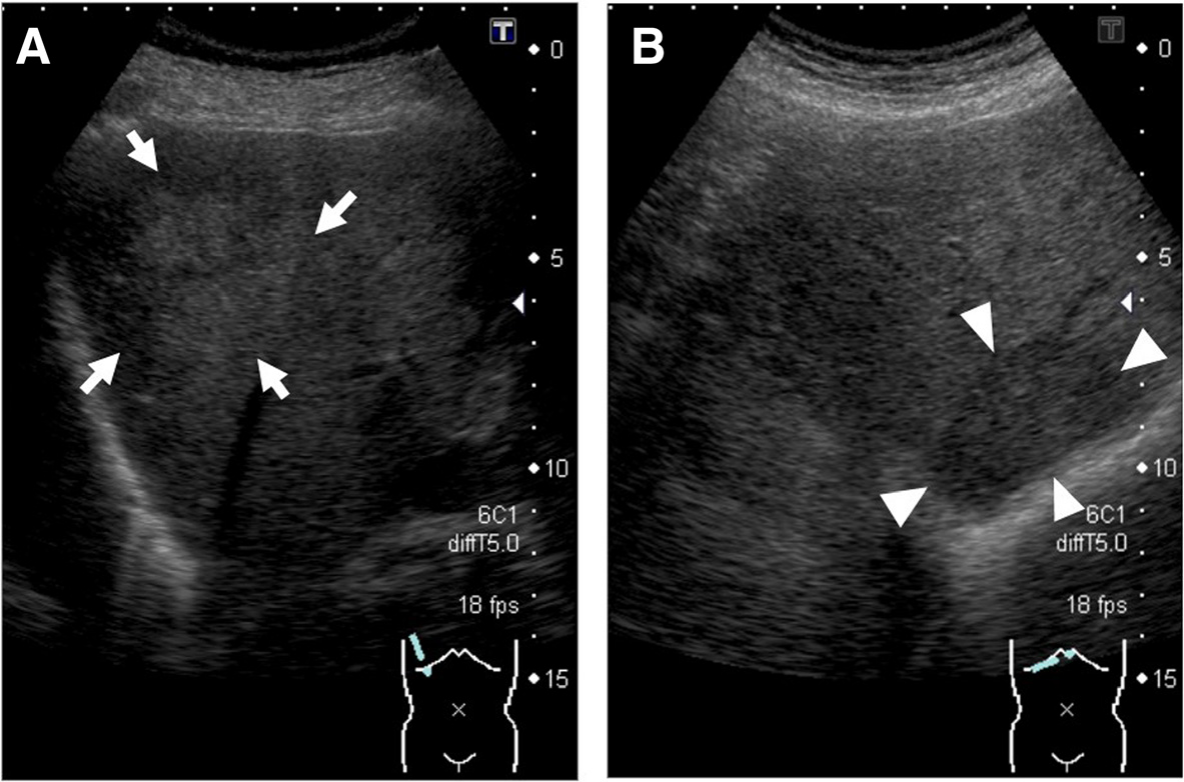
Abdominal ultrasonography shows two hepatic tumors in March 2012. a A high echoic tumor (50×36mm) with an unclear margin was observed in segment 7 (*white arrows*). b A low echoic tumor (30×18mm) with a clear margin was observed in segment 6 (*white arrowheads*)
